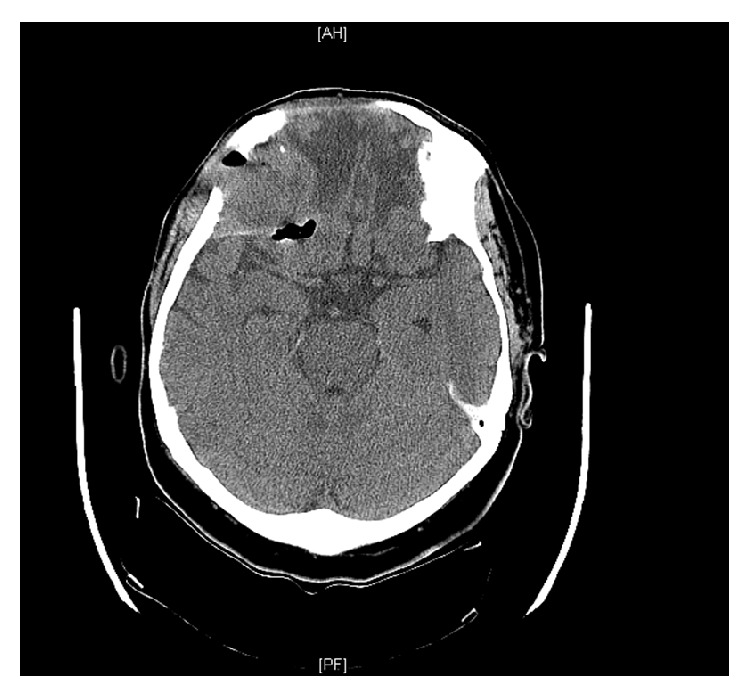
Noncontrasted CT demonstrating brain abscess and pneumocephalus.
